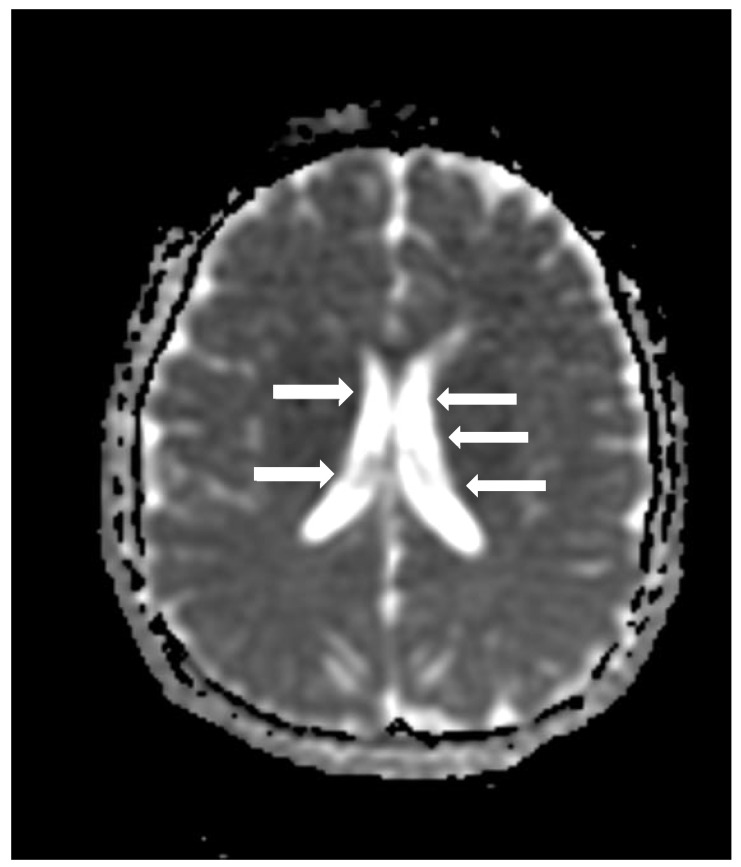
An axial image of the MRI of the skull showing PVNH in both lateral ventricles. Figure legend: white arrows indicate areas of PVNH, visible as discrete ripples of gray matter adjacent to lateral ventricles.
